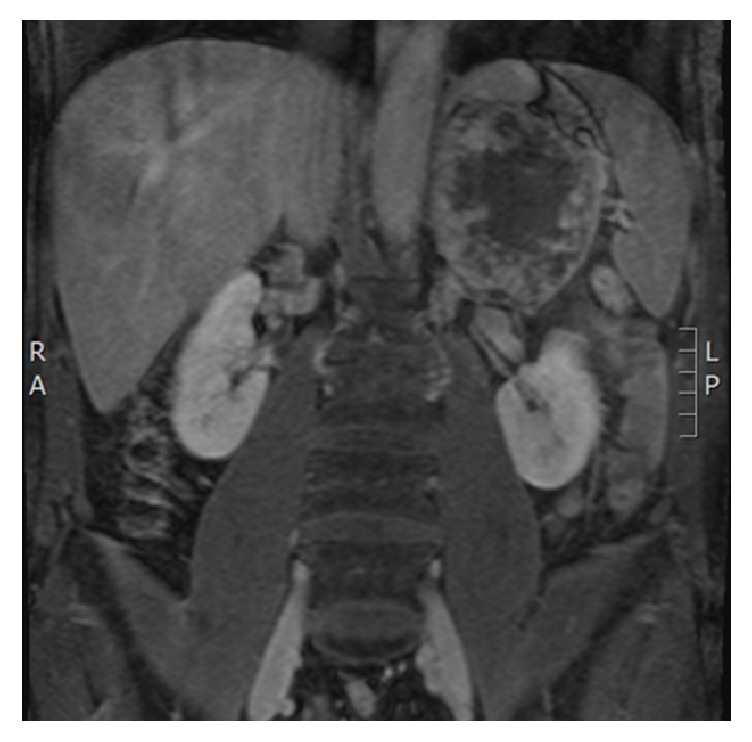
Gadolinium-enhanced T1 abdominal MRI, coronal view, showing a large heterogeneous left adrenal mass.
